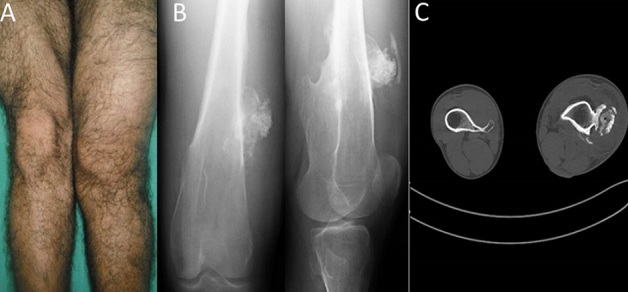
A)Clinical picture showing swelling in the distal third of the left thigh; B) Radiography of the left thigh showing a bony overgrowth; C) CT scan showing pseudo-tumoral aspect of tuberculosis associated with calcifications
